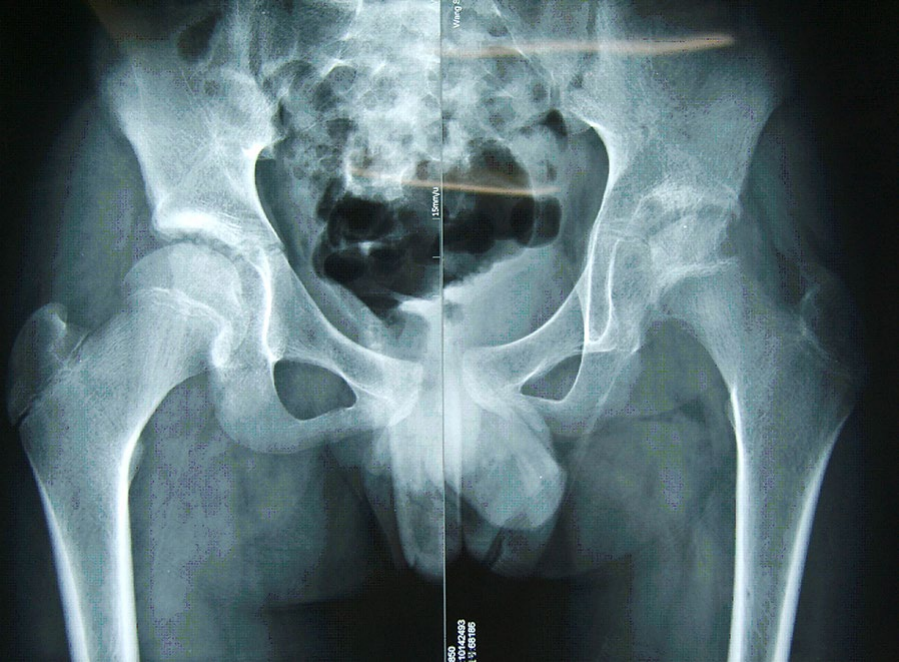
X-ray plain film of bilateral hip joints. The left femoral epiphysis was irregular with bone defect areas at both outer and inner margins; the space of the left hip joint had local stricture; peripheral spindle-shaped soft tissue density shadows were observed. The right acetabulum was thickened and the density was increased
